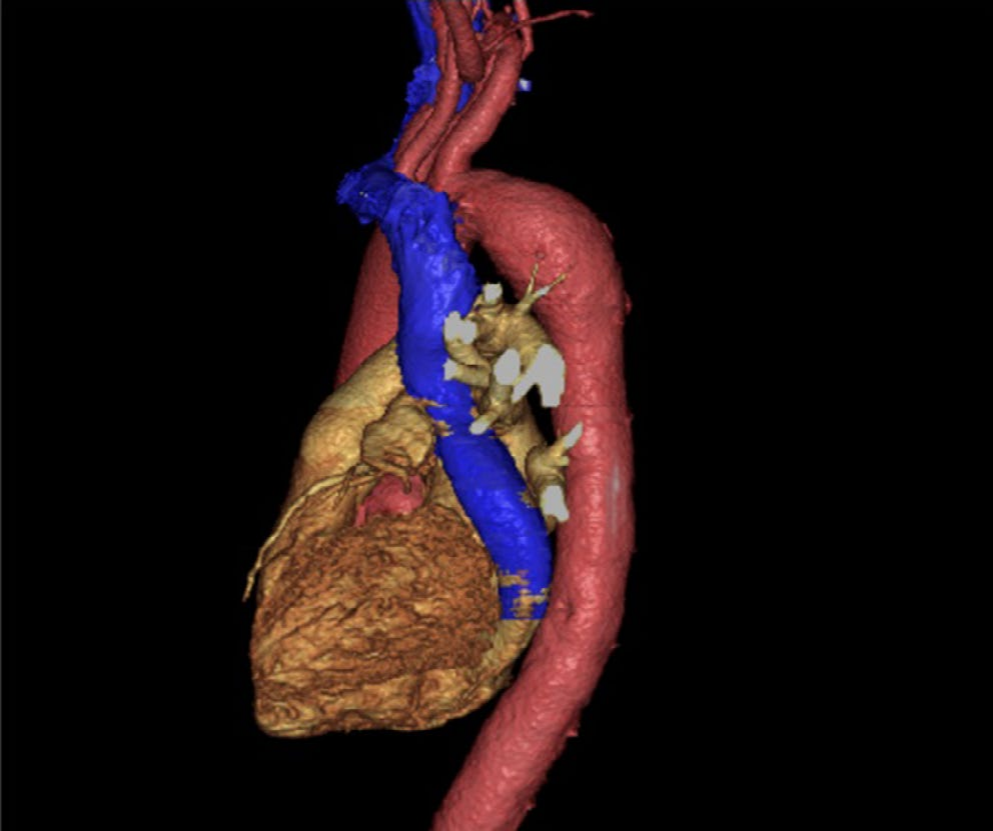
A three‐dimensional CT image (lateral view): A persistent left superior vena cava leading to the right atrium via a dilated coronary sinus. CT, computed tomography
